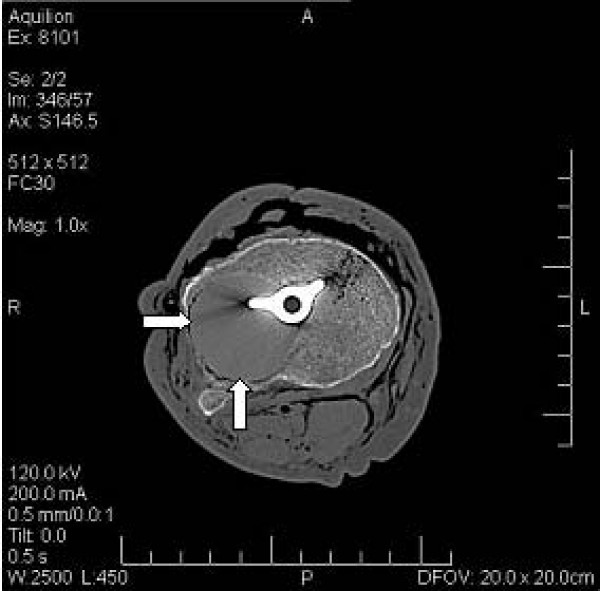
Axial CT scan of tibial osteolytic defect as shown in Figure 1.
